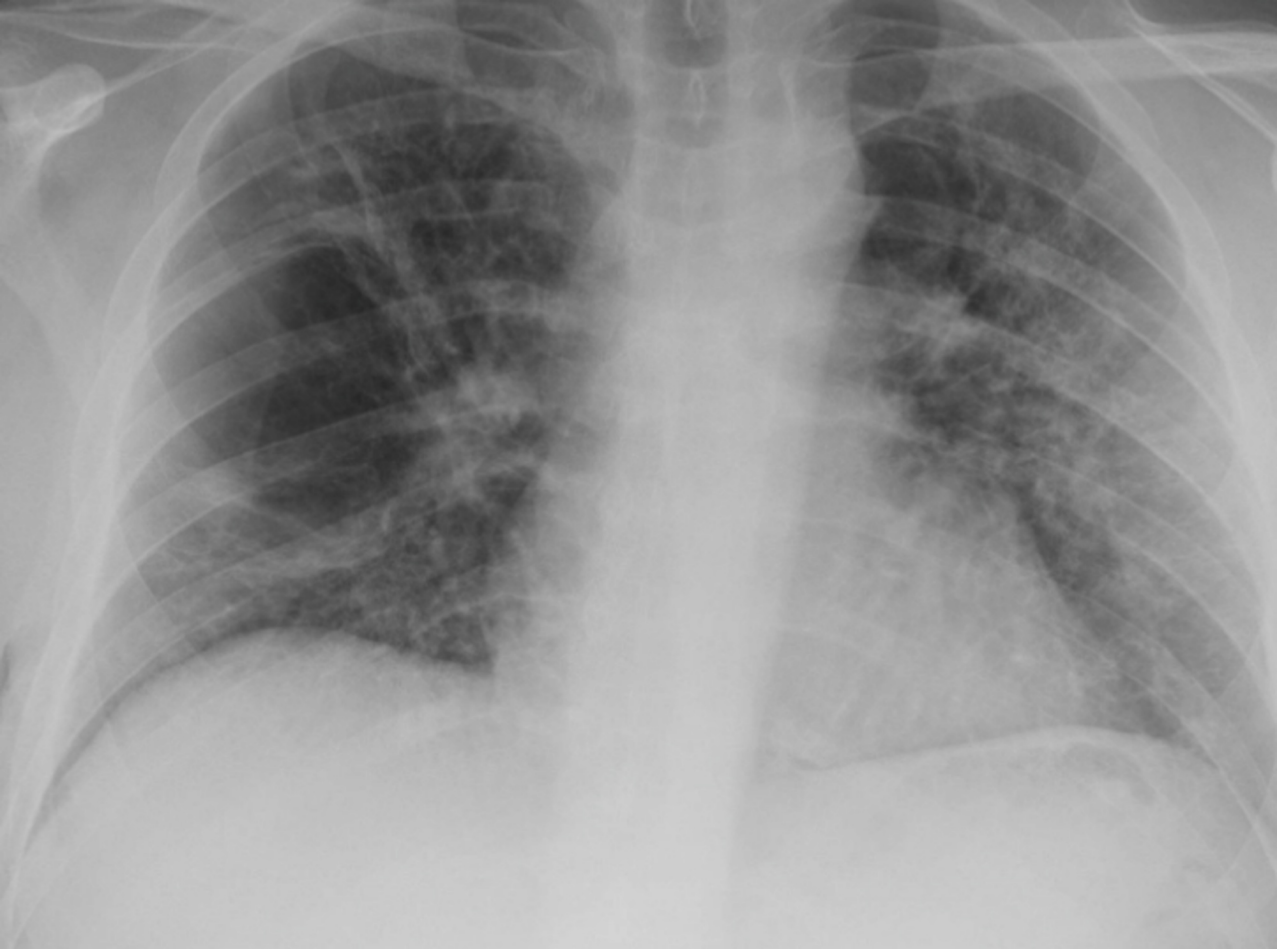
Chest X-ray showing fully re-expanded lungs after chest tube removal on right side.
